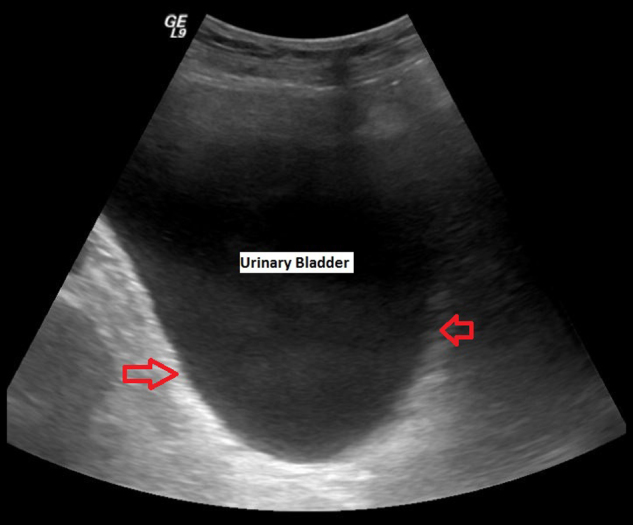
Ultrasound scan of urinary bladder performed in March 2009 (two years after spinal cord injury) showed normal bladder; no bladder wall thickening, diverticula or calculi (right and left arrows)
